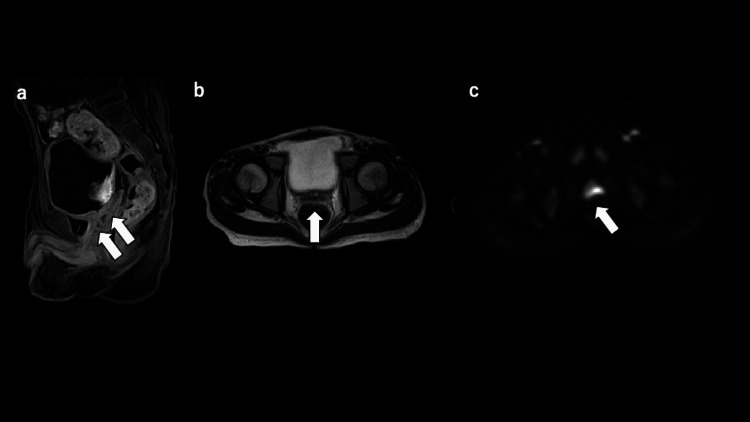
Magnetic resonance imaging. (a) Contrast-enhanced T1-weighted image (sagittal view) revealed peripheral contrast enhancement with internal heterogeneity. (b) The T2-weighted image (axial view) shows a central high-signal and marginal low-signal area. (c) The diffusion-weighted image shows a high signal.
